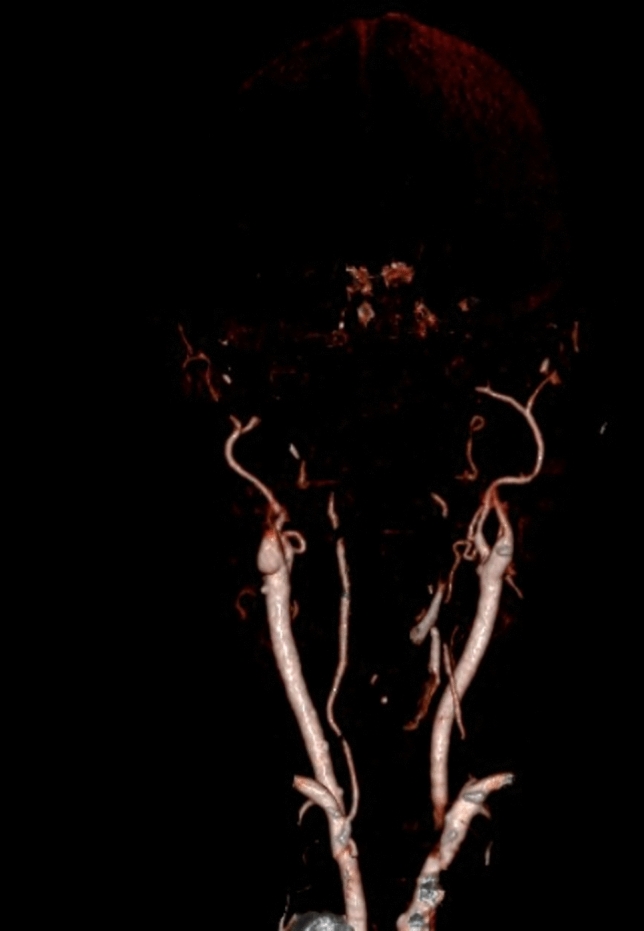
Cerebral computed tomography angiography of Subject 2. Reconstruction showing a typical finding compatible with cerebral death. No contrast filling of both intern carotid arteries after the petrous segment. Both vertebral arteries present no filling after the V3 segment. The extern carotid arteries are bilaterally perfused.
